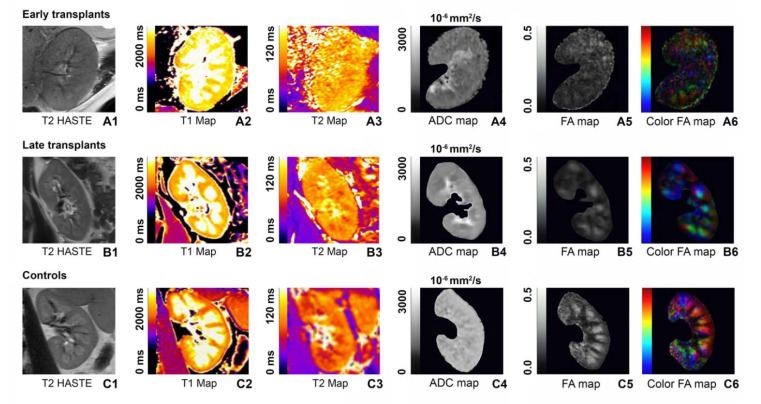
(A1–A6) Standard macroscopic T2-weighted sequence (A1) and images of renal T1 (A2) and T2 maps (A3) as well as ADC (A4) and FA maps (A5,A6) for an early transplant. (B1–B6) Images of a late transplant (half a year since transplantation). (C1–C6) MR images of a control with a healthy native kidney. Corresponding color bars are presented on the left side of each map, with 0–2000 ms for T1 maps, 0–120 ms for T2 maps, 0–3000 × 10^−6^ mm^2^/s for ADC maps, and 0.0–0.5 for FA maps. Generally, lighter colors/greyscales indicate higher values. As one can see from the above color-coded images, T1 and T2 relaxation times are highest in the newly transplanted kidney (A1–A3) and lowest in the healthy native kidney (C1–C3). In contrast, the ADC and especially the FA map for the newly transplanted kidney shows the lowest ADC and FA values with only moderate differentiation between cortex and medulla (A4,A5), while ADC and FA values are higher for the older stable allograft (A4,B5) and highest for the healthy native kidney (C4,C5).
